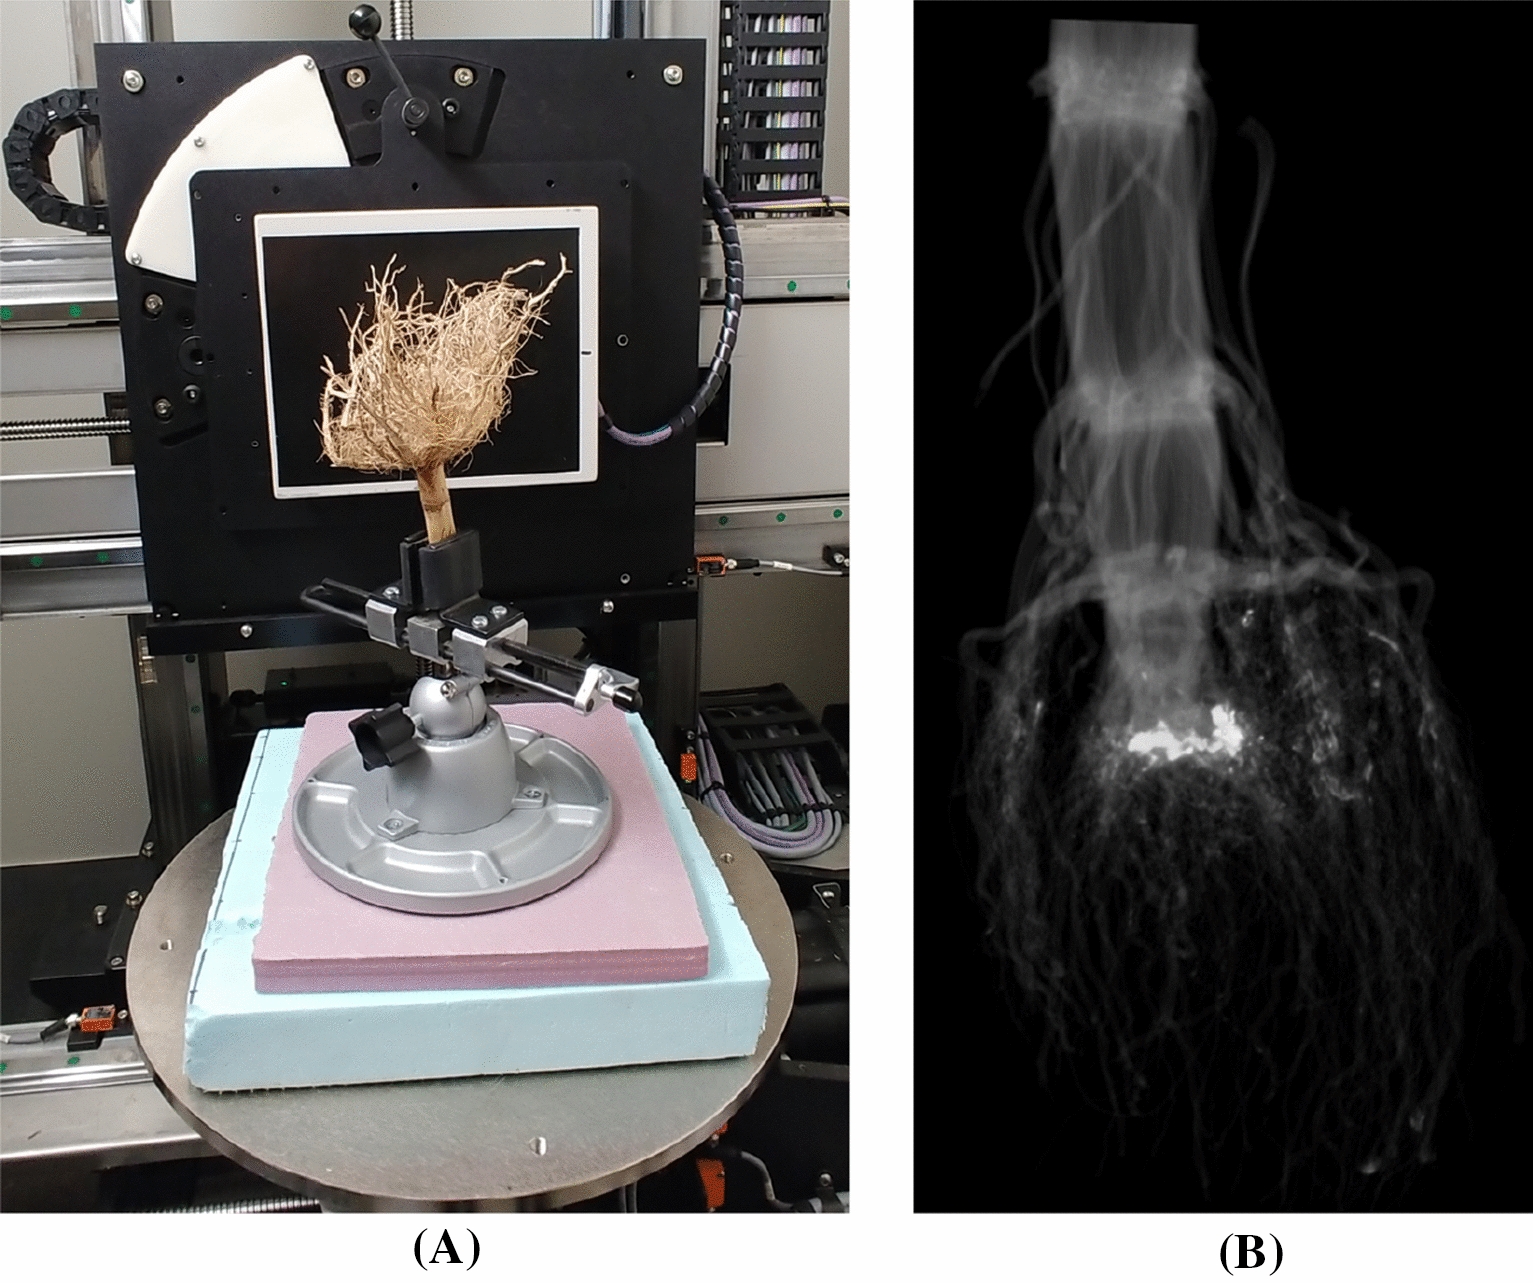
X-ray CT imaging of root crowns. A Each maize root crown was clamped and placed on a turntable, which was rotated for 3 min while radiographs were collected at a rate of 10 frames per second. B efX-CT software was used to reconstruct a 3D grayscale image from the scan, which is represented as a stack of image slices perpendicular to the z-axis
